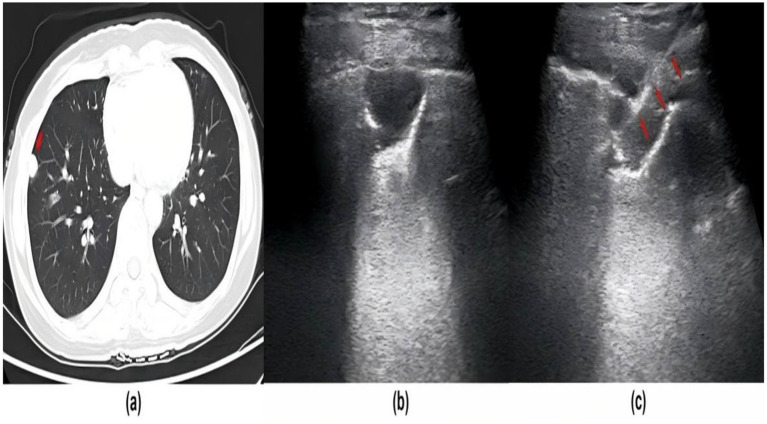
Ultrasound-guided lung puncture. (a) CT image of the peripheral pulmonary lesion (red arrow) located at the right upper lobe. (b) Baseline ultrasound showed the irregular and hypoechoic lesion (red arrow). (c) A 16-G needle was used to perform biopsy for the lesion. The needle tract (red arrow) could be seen on ultrasound.
